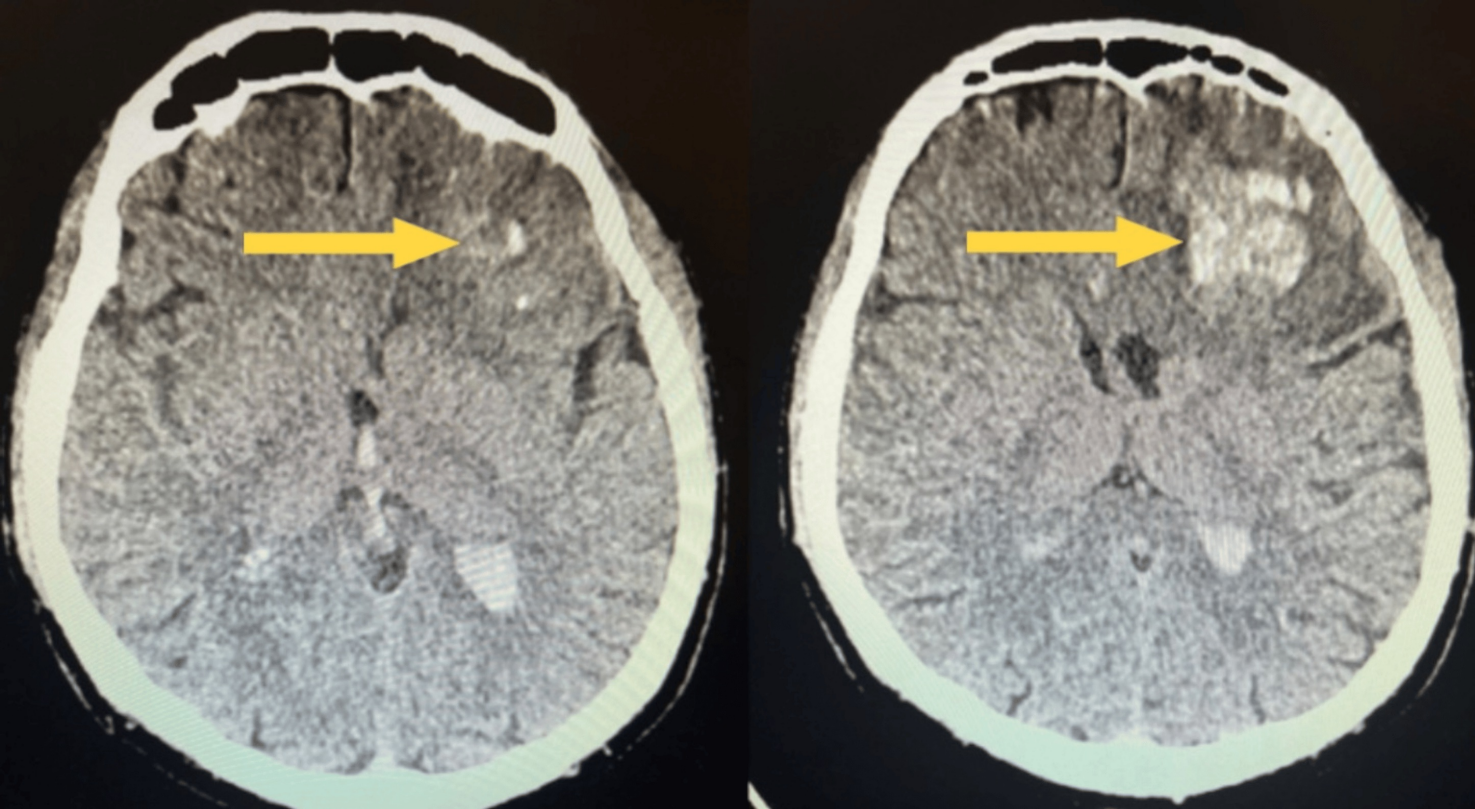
CT scan of the brain performed on the first day of hospitalization Non-contrast CT brain scan showing a large left frontal intracerebral hematoma with intraventricular and subarachnoid extension (arrow).
